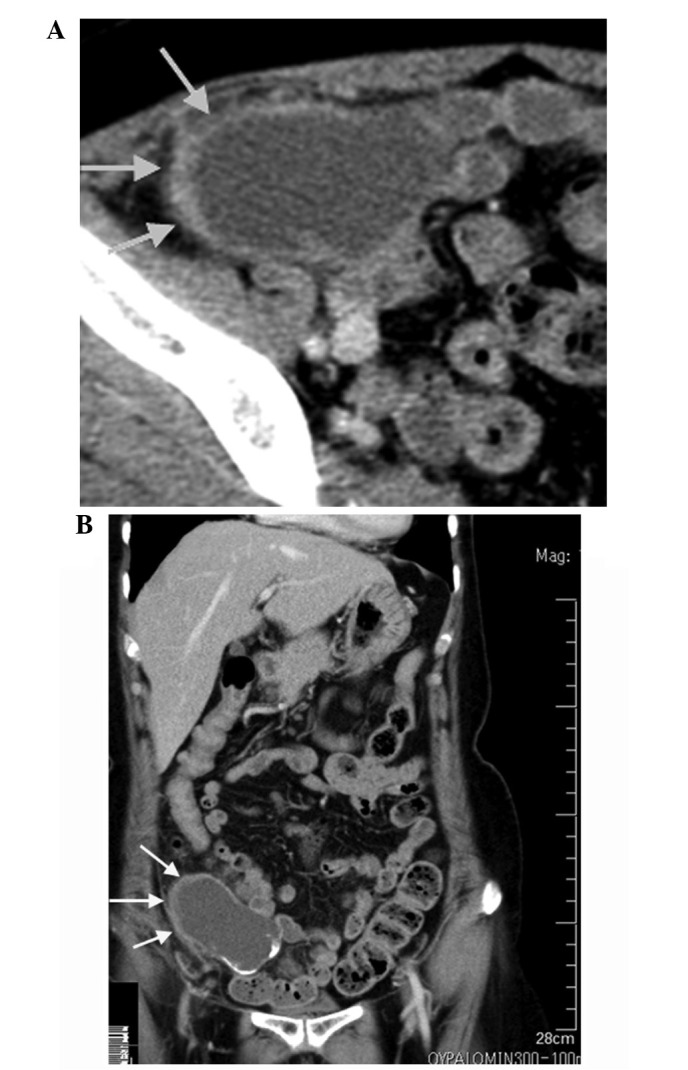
Abdominal computed tomography (CT) scans obtained in August 2012. Axial view (A) and coronal view (B) show no change in the size of the cystic mass at the site of the appendix. Thickening of the mass wall on the appendicular ostium was visible, with contrast enhancement at the corresponding site (arrow). No projection was visible in the mass cavity.
